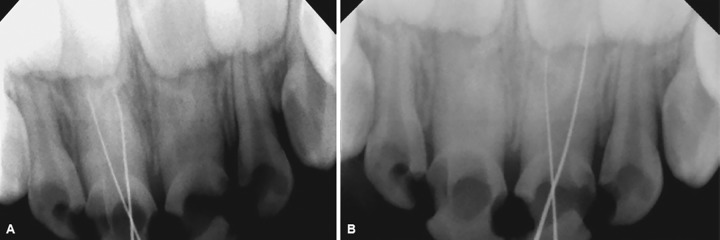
(A) Working length determination radiograph with 51; and (B) working length determination radiograph with 61
